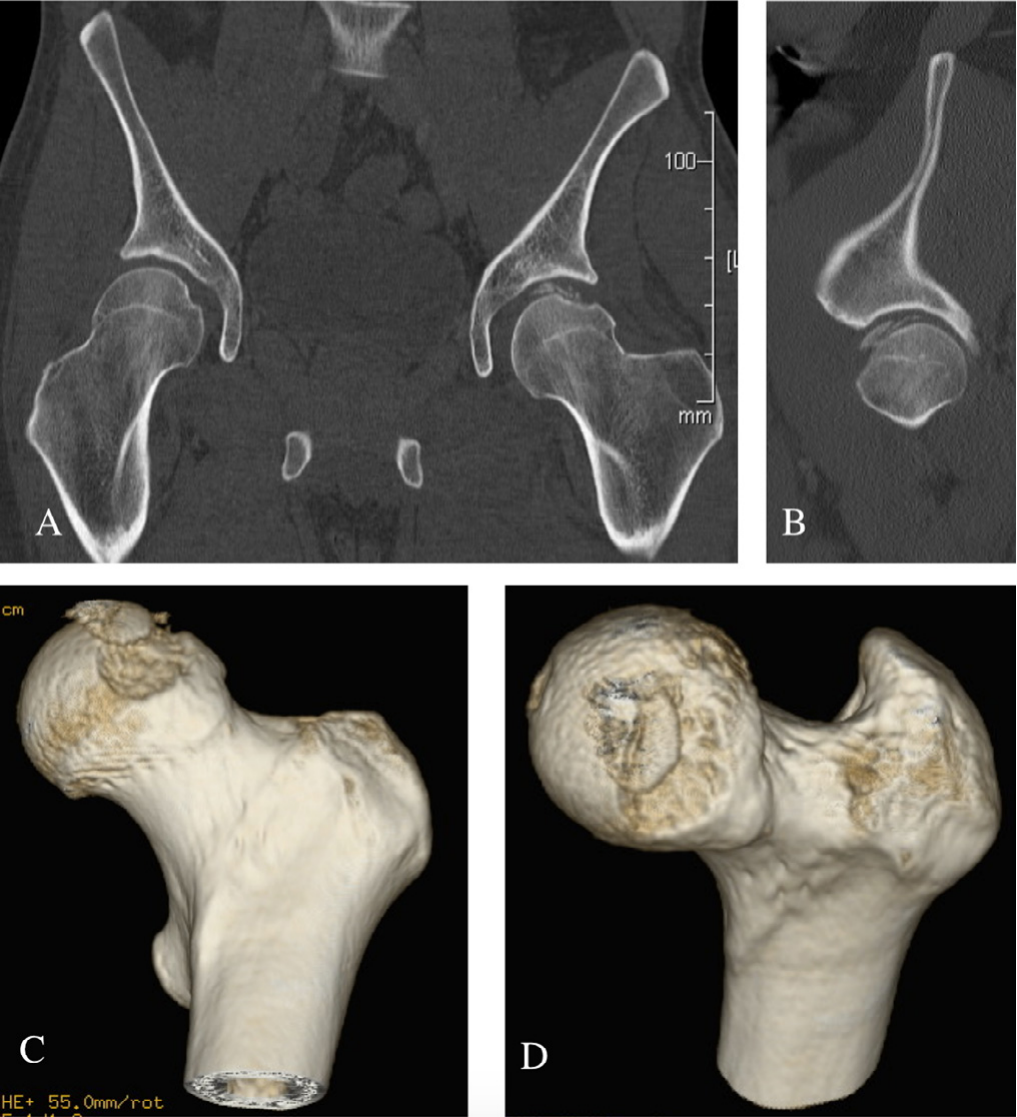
Patient 1: Post Reduction Coronal slice (A), Sagittal Slice (B) and 3D reconstruction (C,D) CT demonstrating femoral head impaction and osteochondral defect in weight bearing portion of femoral head.
